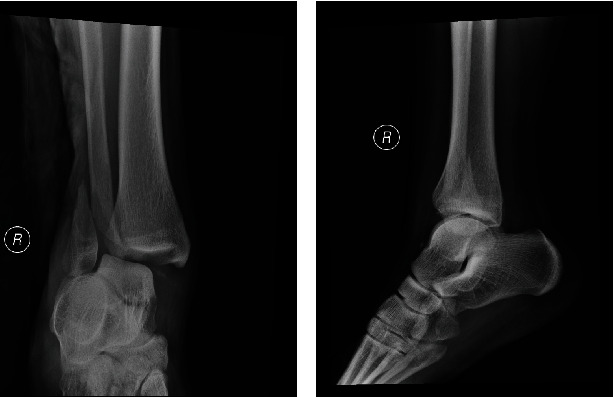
(a, b) X-ray of the right ankle AP and lateral view of the patient.
